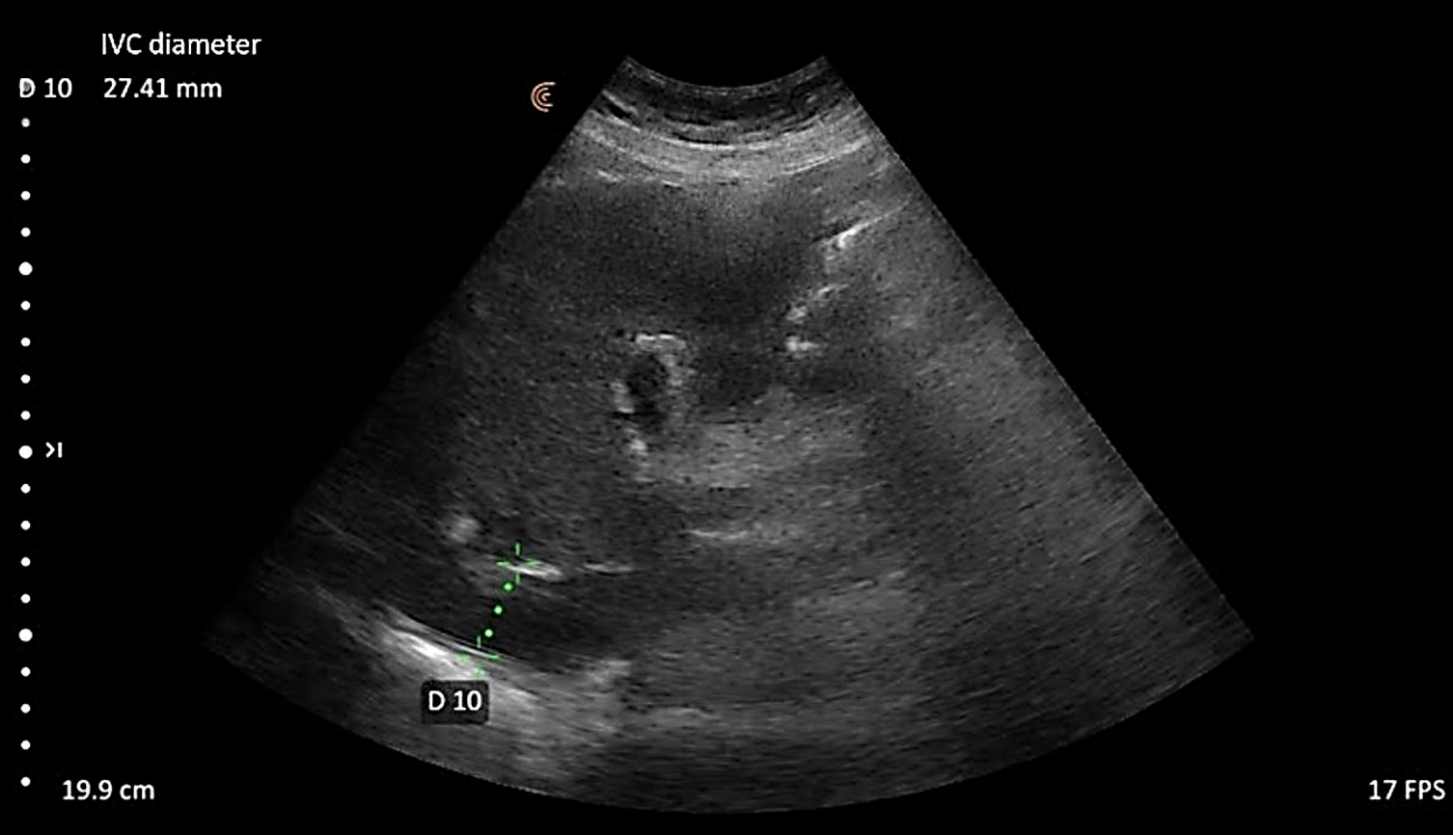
Measurement of IVC diameter on abdominal ultrasound.
